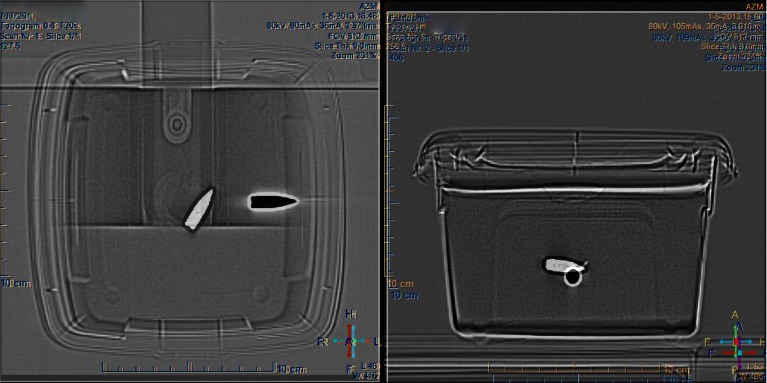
Top view (*left*) and lateral view (*right*) of a CT of gelatine 3. The projectile before exposure, the magnetic field of the MRI is shown in *white*; the position of the projectile after exposure to the MRI is shown in *black*. A clear displacement of the projectile has occurred along the direction of the main axis of the static MRI field in combination with a rotation of the projectile
